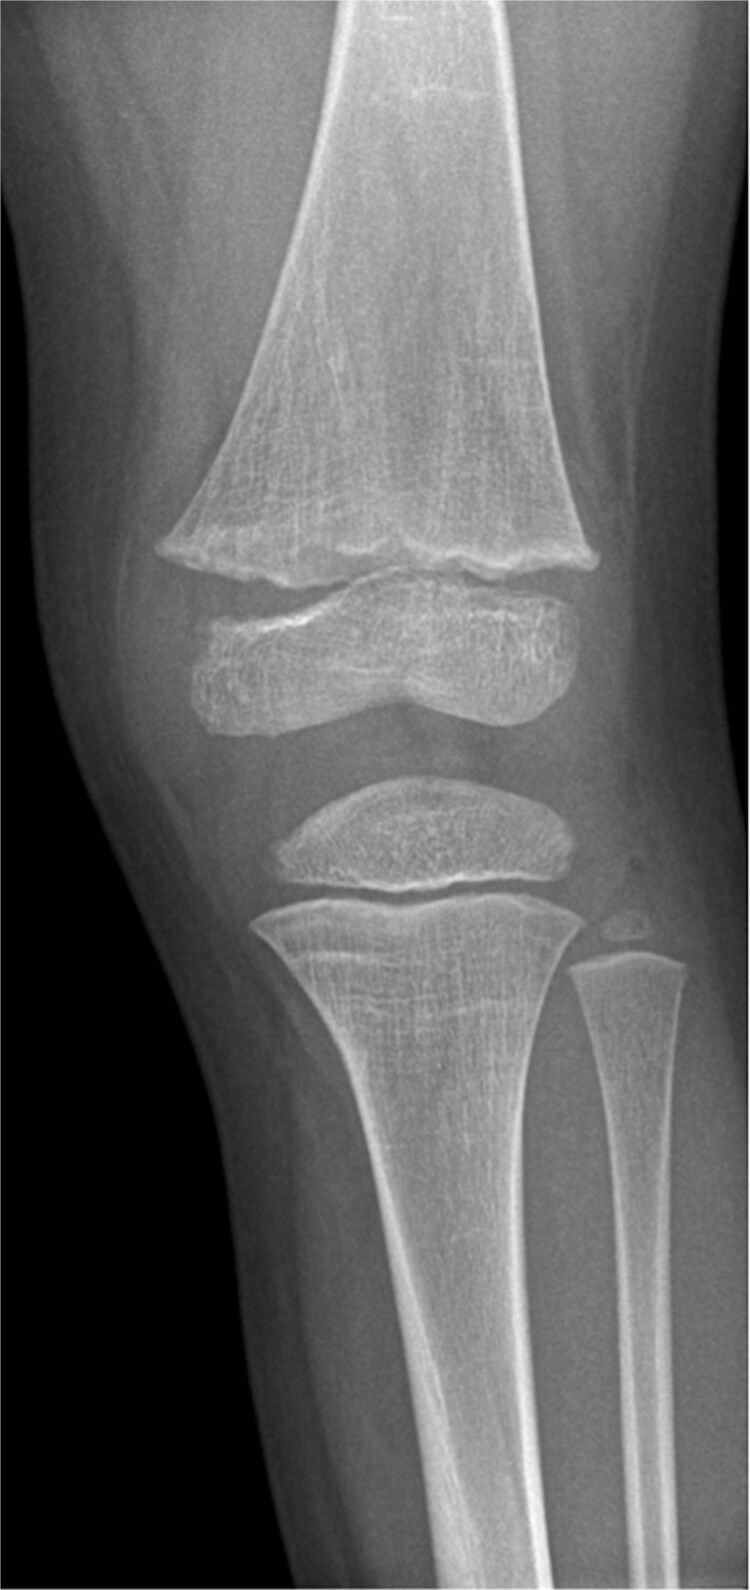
Radiograph demonstrated generalized changes of rickets with flaring and cupping of the metaphyses.
